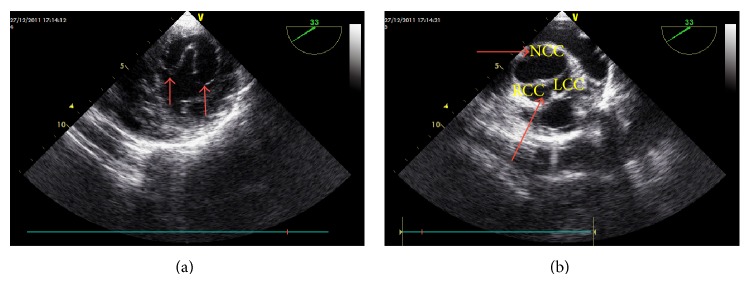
Modified section of mitral valve and short axis view of the bicuspid aortic valve with fusion of the left and right coronary cusps (LCC and RCC) in transesophageal echocardiography.
